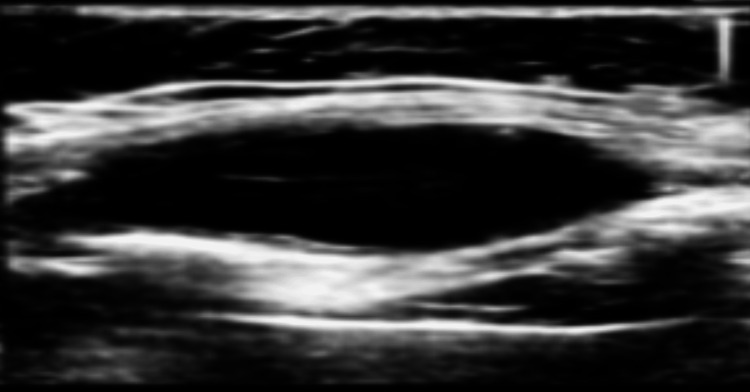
Ultrasound of the chest wall abscess. Ultrasound images of the right chest wall demonstrate a well-defined hypoechoic collection with a thick wall along the lower rib, measuring approximately 4.0 × 1.4 × 2.8 cm (estimated volume ~8.8 mL). The adjacent rib shows cortical irregularity, suggestive of underlying osseous involvement.
